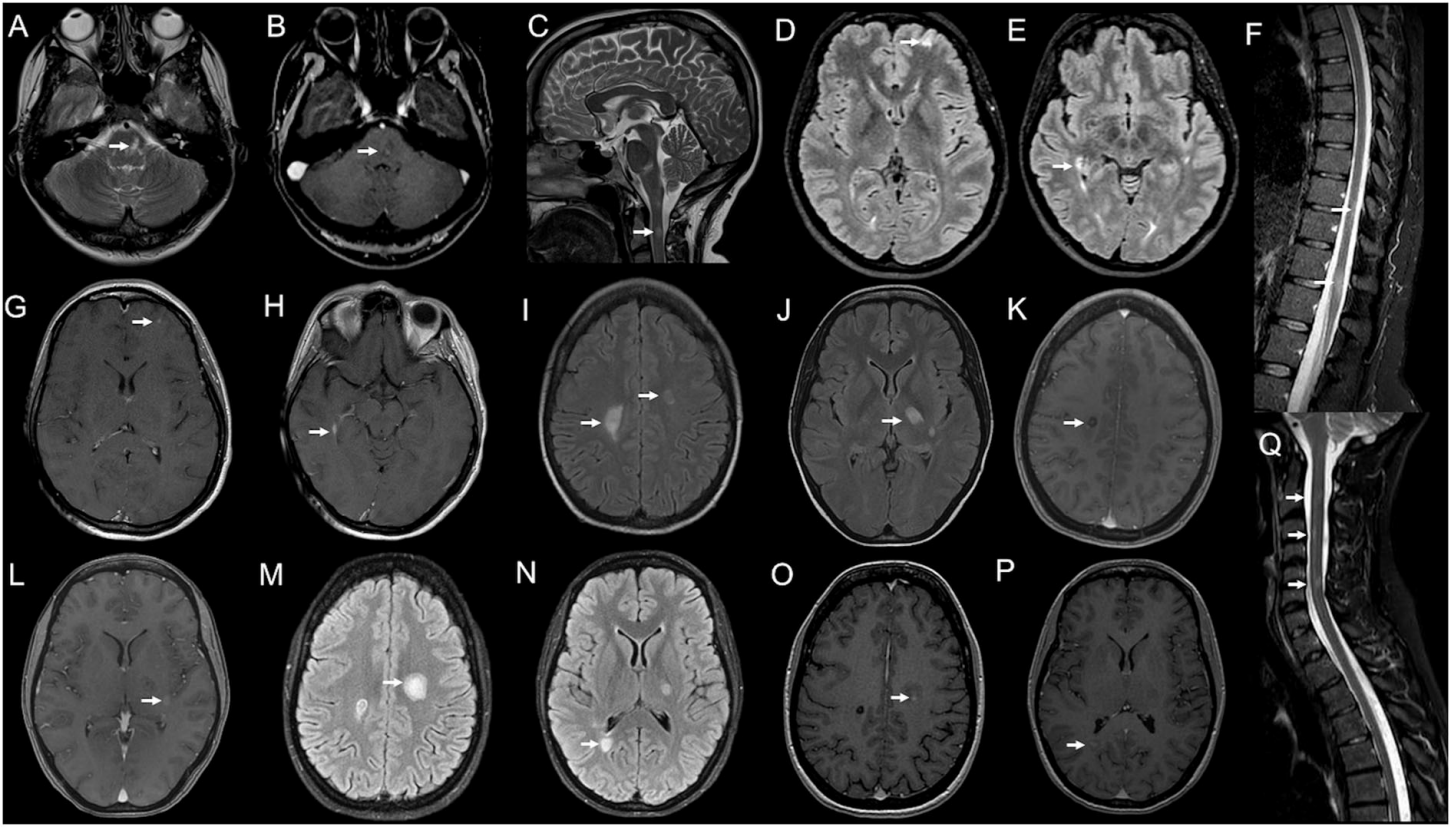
Serial brain and spinal cord MRI scans in Case 3 (A–H) and Case 4 (I–Q). Case 3: (A) Brain MRI performed 25 days after BNT162b2 second dose, showing a T2-weighted hyperintensity (arrow) in the left paramedian mid-pons with blurred enhancement on T1-weighted images [(B); arrow] and a non-enhancing spinal lesion at C2–C3 level [(C); arrow]. (D–H) 2-months MRI follow-up showing two new brain lesions—one juxtacortical in the left frontal hemisphere [(D); arrow] and one periventricular abutting the right lateral ventricle occipital horn [(E); arrow], both with contrast-enhancement [(G,H); arrows] and two spinal lesions at the dorsal level [(F); arrows], with no contrast enhancement (not shown). Case 4: (J–L) Brain MRI obtained 10 days after BNT162b2 second dose, revealing T2-weighted hyperintensities in both centra semiovale (CSO) [(I); arrows] and left thalamus/posterior limb of the internal capsule (IC) [(J); arrow]; lesions in the right CSO [(K); arrow] and left IC [(L); arrow] showed blurred contrast enhancement on T1-weighted images. (M–P) 20-days MRI control showing the enlargement of the left CSO lesion [(M); arrow] and a new lesion in the right peritrigonal area [(N), arrow], both with gadolinium-enhancement on T1-weighted images [(O,P); arrows]. (Q) Spinal cord MRI—obtained 10 days after the brain exam—showing three blurred areas at C2, C3, and C5–C6 levels on STIR sequences, with no enhancement (not shown).
